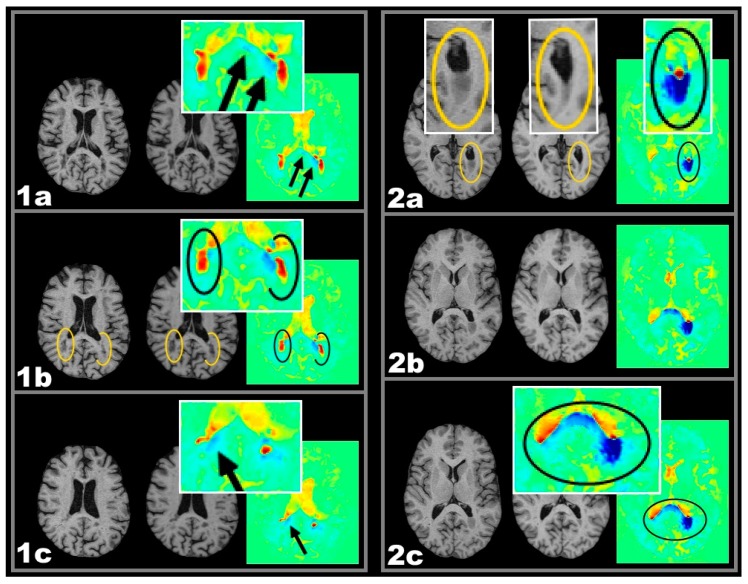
One year follow-up T1w MRI and respective colour coded VGM maps demonstrating different types of volume change. Important detail is highlighted in elipses. Two exemplary cases with active lesions and spatially related callosal volume decrease: Case 1 CEL, Case 2 CSL. From left to right: MRI-1, MRI-2, and VGM. Case 1 CEL: (1a–1c) Three slices showing bilateral CELs (ellipses in 1b) close to the posterior horns of the lateral verntricles while callosal shrinkage is observed (arrows in 1a and 1c) seen as blue colors; Case 2 CSL: (2a–2c) Three slices showing a periventricular CSL at the posterior horn of the left lateral ventricle. Adjacent callosal fibers show a volume reduction in the splenium of the corpus callosum. This callosal volume reduction extends to the contralateral hemisphere (2c). Important detail is highlighted in circles.
